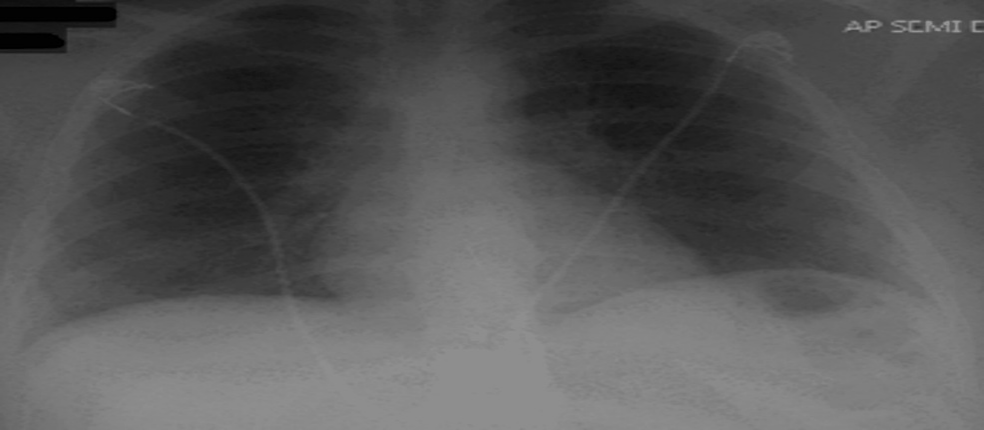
CXR with resolved bilateral opacities. Chest X-ray obtained the day before the patient was discharged shows a significant decrease in bilateral opacities and infiltrates after treatment with steroids and antibiotics.
